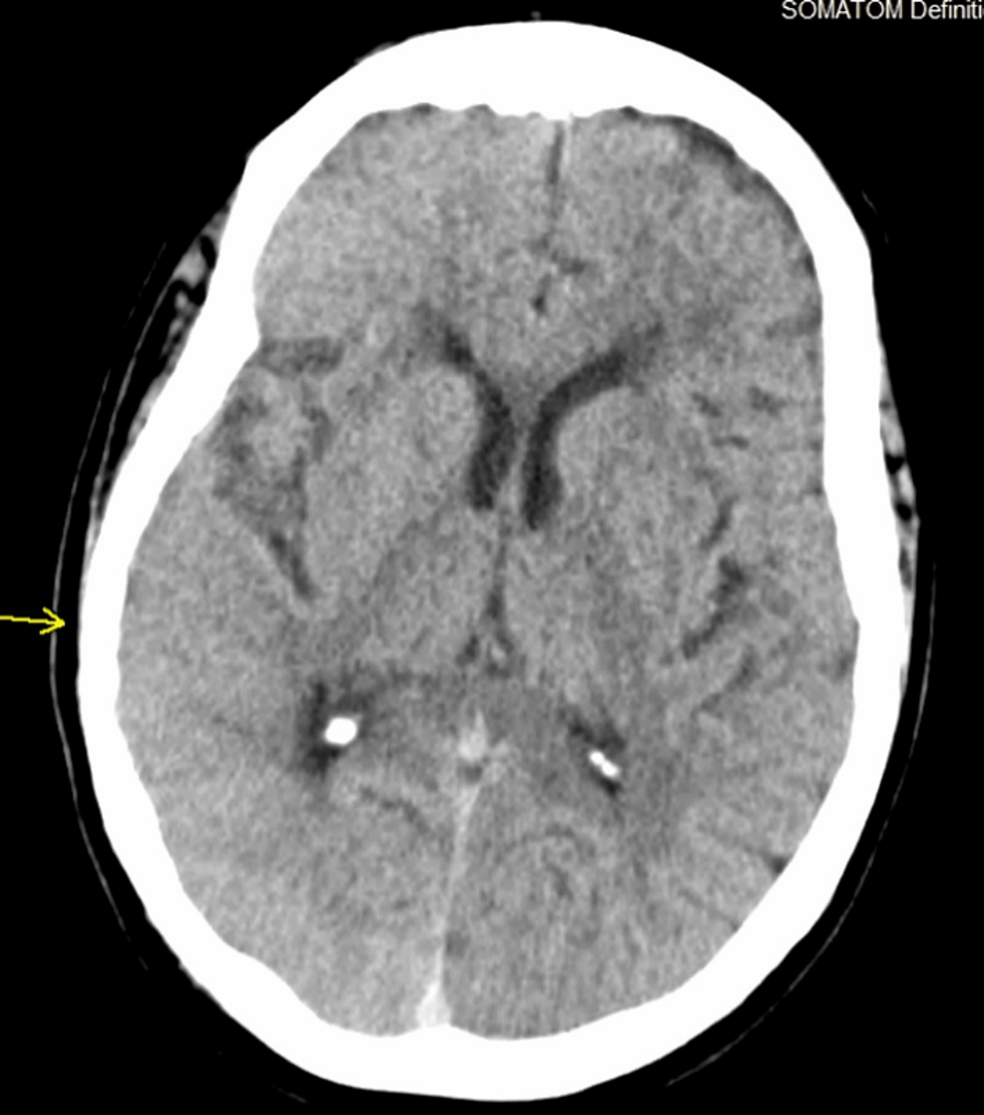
CT scan of the head in the immediate perioperative period after dedicated catheter angiogram study.
